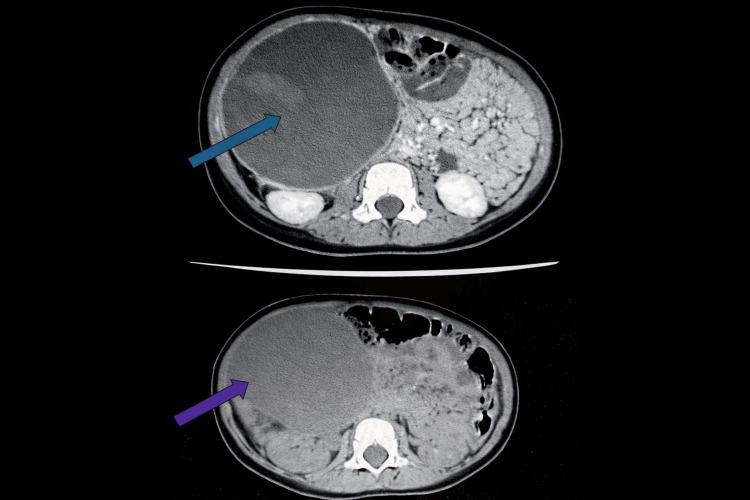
CT of the abdomen and pelvis with contrast (top, blue arrow), and without contrast (bottom, purple arrow) shows a large well-circumscribed hypodense cystic lesion in the right subhepatic region associated with mass effect on liver, gallbladder, bowel, and abdominal wall.
